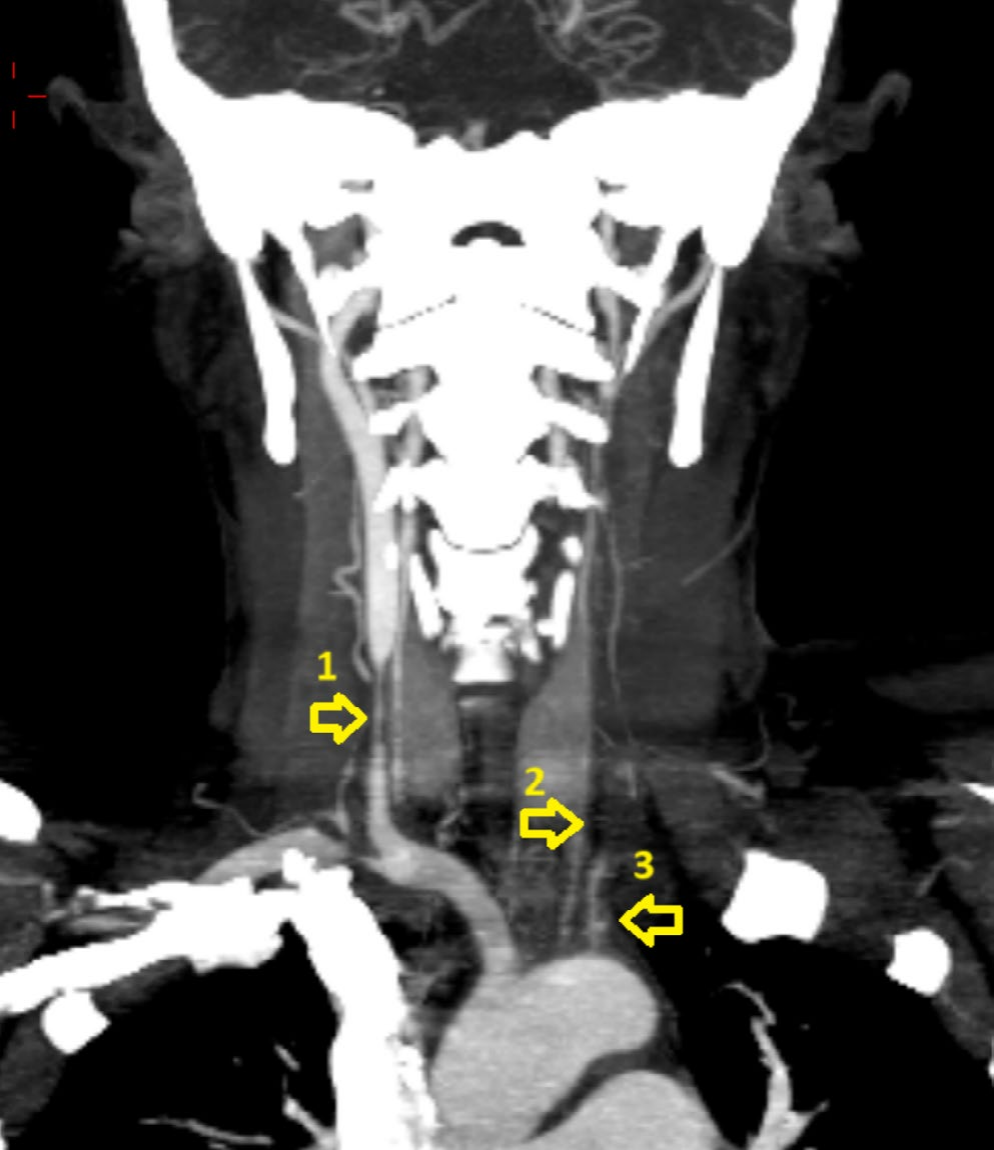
CT‐ Coronal view demonstrating stenosis of all arteries arising from arch of aorta as indicated by arrows. 1: Stenosis of right common carotid artery with post‐stenotic dilation, 2: Left common carotid artery stenosis along its entire course, 3: Left sub‐clavian artery stenosis.
